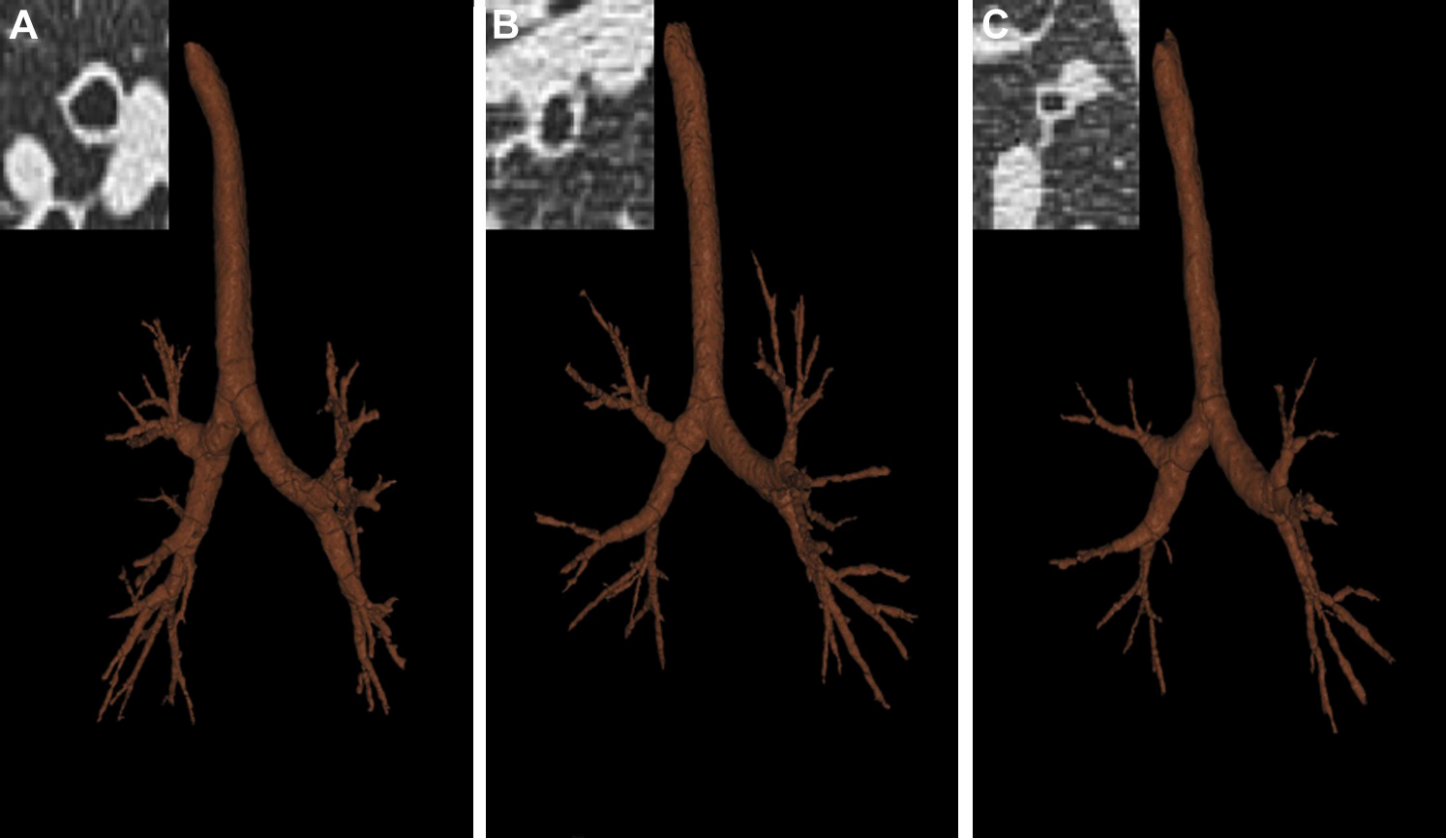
Proximal airway remodeling in asthma phenotypes. Pictures of segmented airway tree and RB1 CT cross-section *(insets)* of asthmatic patients from cluster 1 (A), cluster 2 (B), and cluster 3 (C) are shown.
